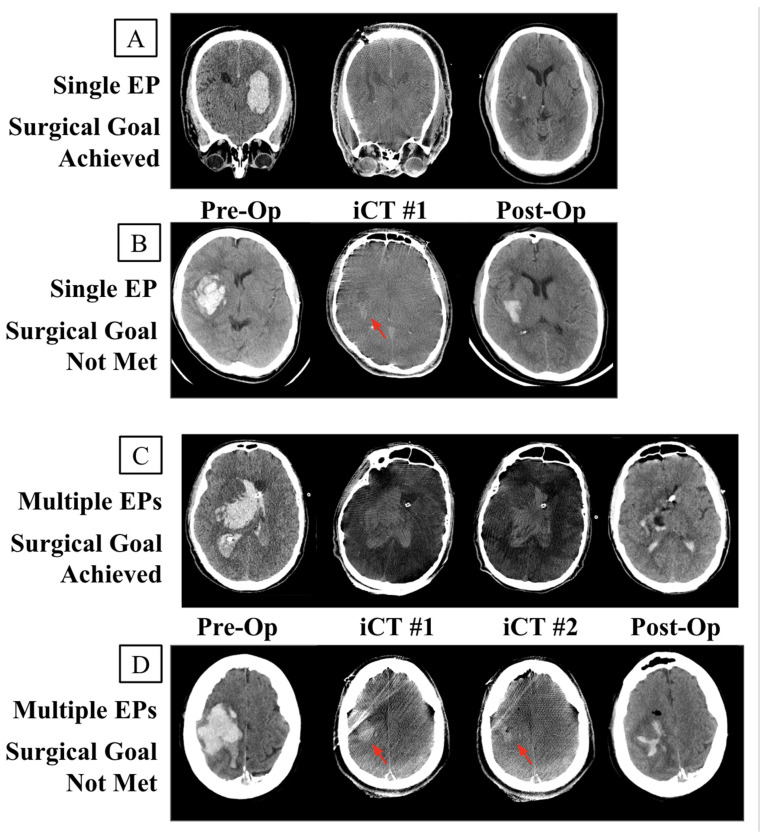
Intraoperative (iCT) and postoperative CT of each case type. (A) Successful surgical goal achieved upon the completion of the original EP. (B) Surgical goal not achieved but no secondary EPs conducted due to additional complications. (C) Surgical goal not achieved at the time of the initial iCT but achieved following multiple EPs. (D) Multiple EPs attempted to rescue the surgical goal without success. Red arrow identifying residual bleed.
